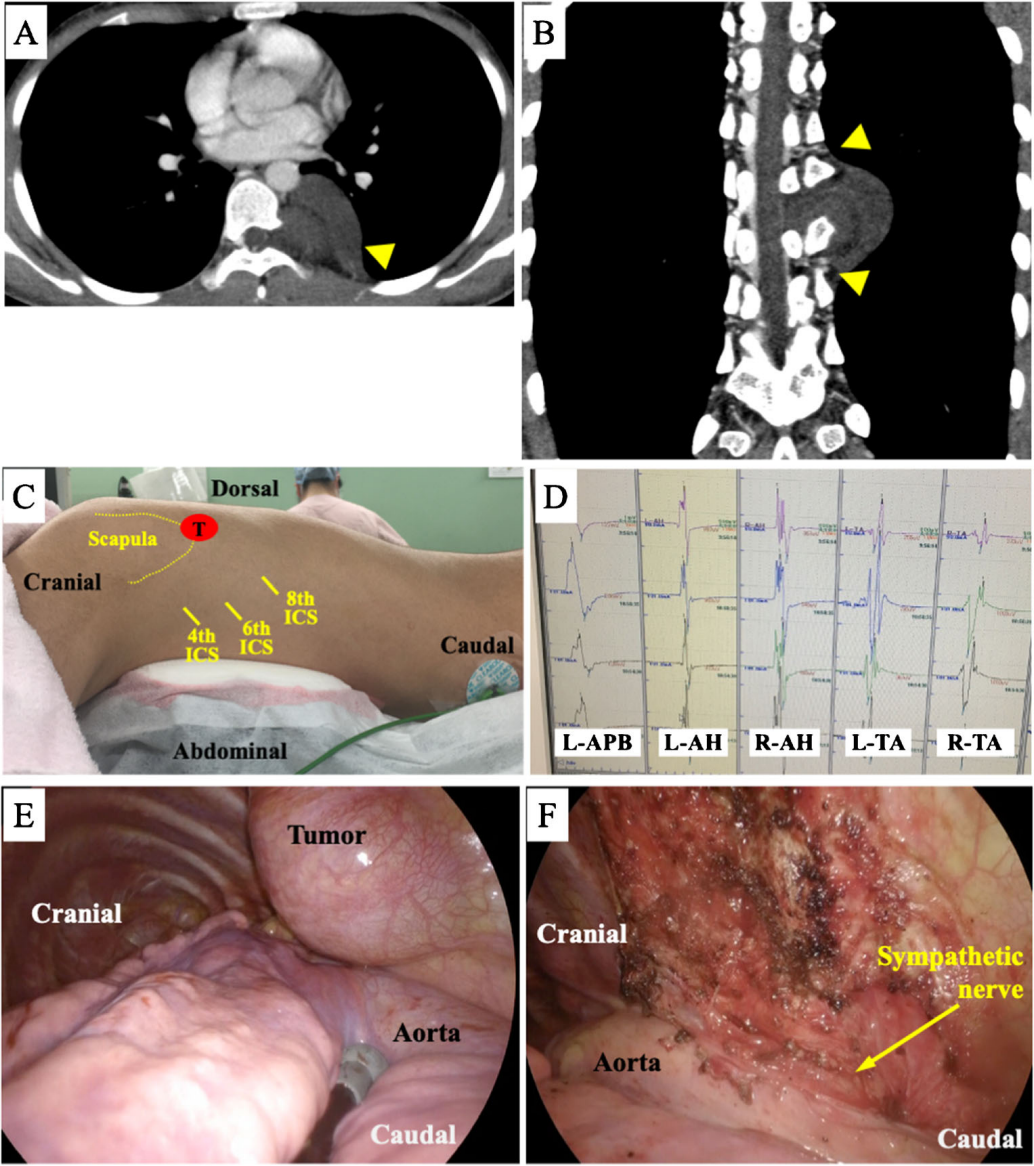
Images of patient 2. A The tumor was located in the left posterior mediastinum and was suspected to extend to the spinal canal through the seventh thoracic (Th7) intervertebral foramen on computed tomography (CT). B The AKA ran through the sixth thoracic (Th6) and eighth thoracic (Th8) intervertebral foramina (arrowheads). C Prone position during VATS and the locations of the three thoracic ports. These positions did not change during the operation. D Motor evoked potentials (MEPs) were used intraoperatively to monitor the descending motor pathway. Intraoperative deterioration of MEPs was not observed during the operation. *L* left, *R* right, *APB* abductor pollicis brevis, *AH* abductor hallucis, *TA* tibialis anterior. E and F Intraoperative photographs showing that both the lung and aorta descended anteriorly with gravity, which produced good surgical fields and kept the forceps from contacting the aorta
